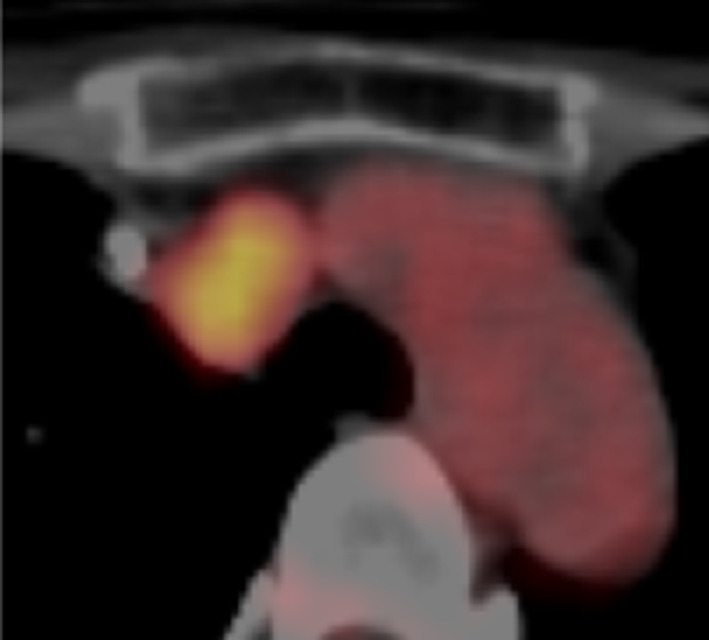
^18^F-FDG-PET/CT. The nodule was the only positive area in the whole body (SUVmax: 4.71)
